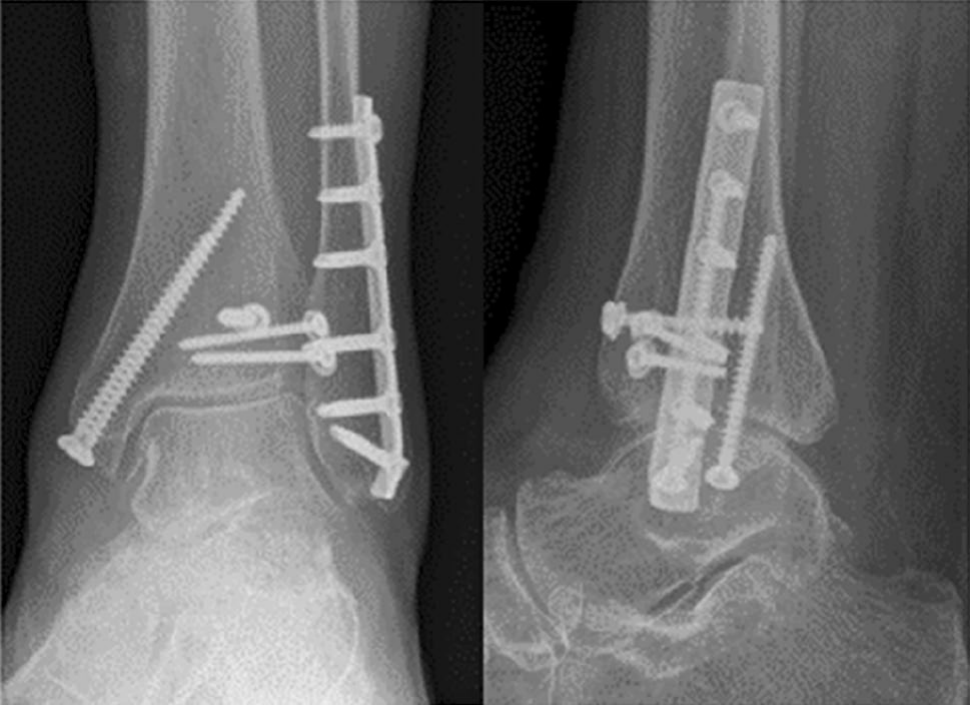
Postoperative anteroposterior and lateral radiographs following open reduction and screw fixation of the anterolateral distal tibia and medial malleolus and plate fixation of the lateral malleolus (same patient in Figs. 1,2)
